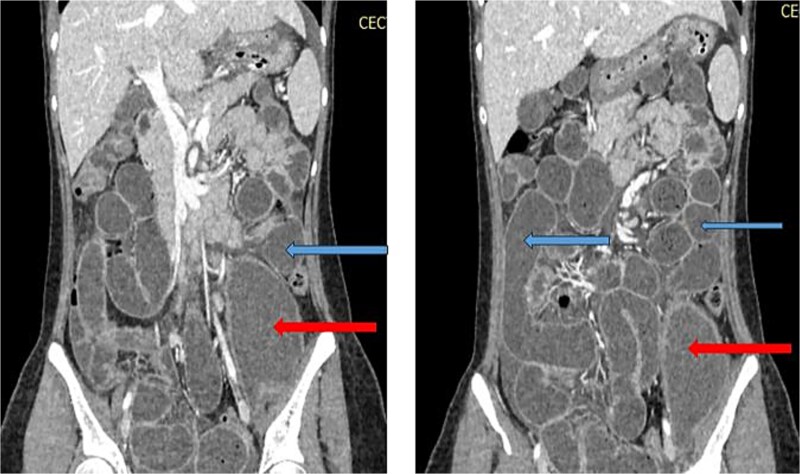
CT abdomen/pelvis with contrast, upper arrows showing dilated small bowel loops and lower arrrows showing collection in left psoas muscle.
